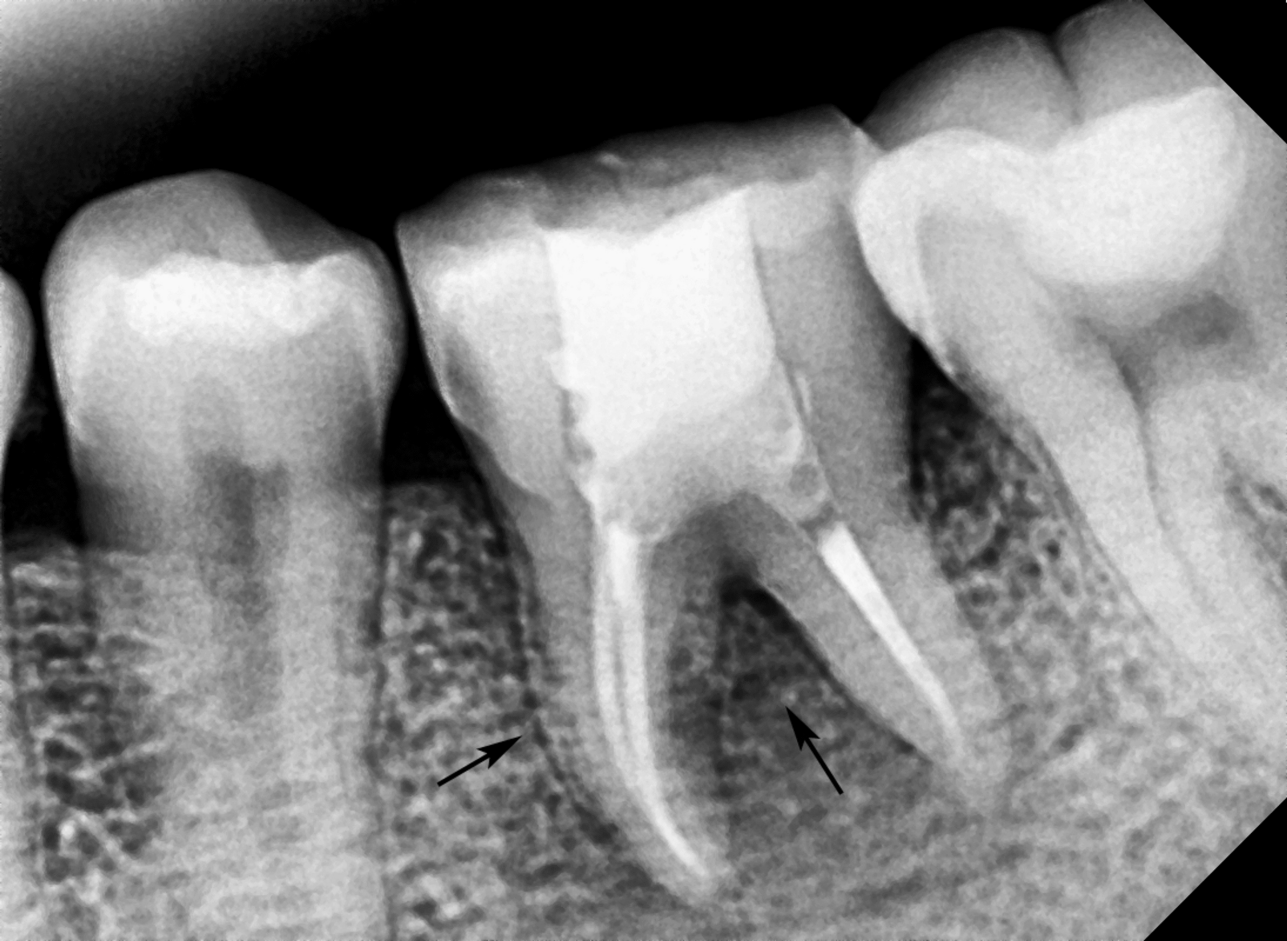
Postoperative intraoral periapical radiograph after six months showing root canal-treated autotransplanted tooth, healed periapical area with periodontal ligament space (black arrows).
